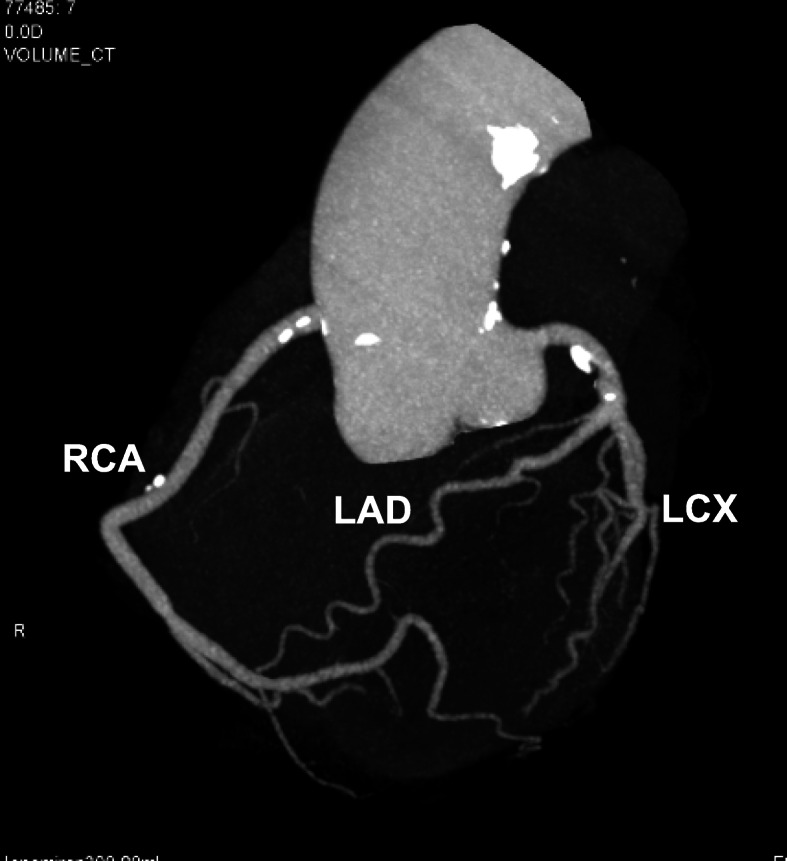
Coronary computed tomography (CT) angiography. Coronary CT angiography revealed no significant stenosis of coronary arteries although dispersed small spotty calcifications were noted. LCX, left circumflex artery; LAD, left anterior descending artery; RCA, right coronary artery
